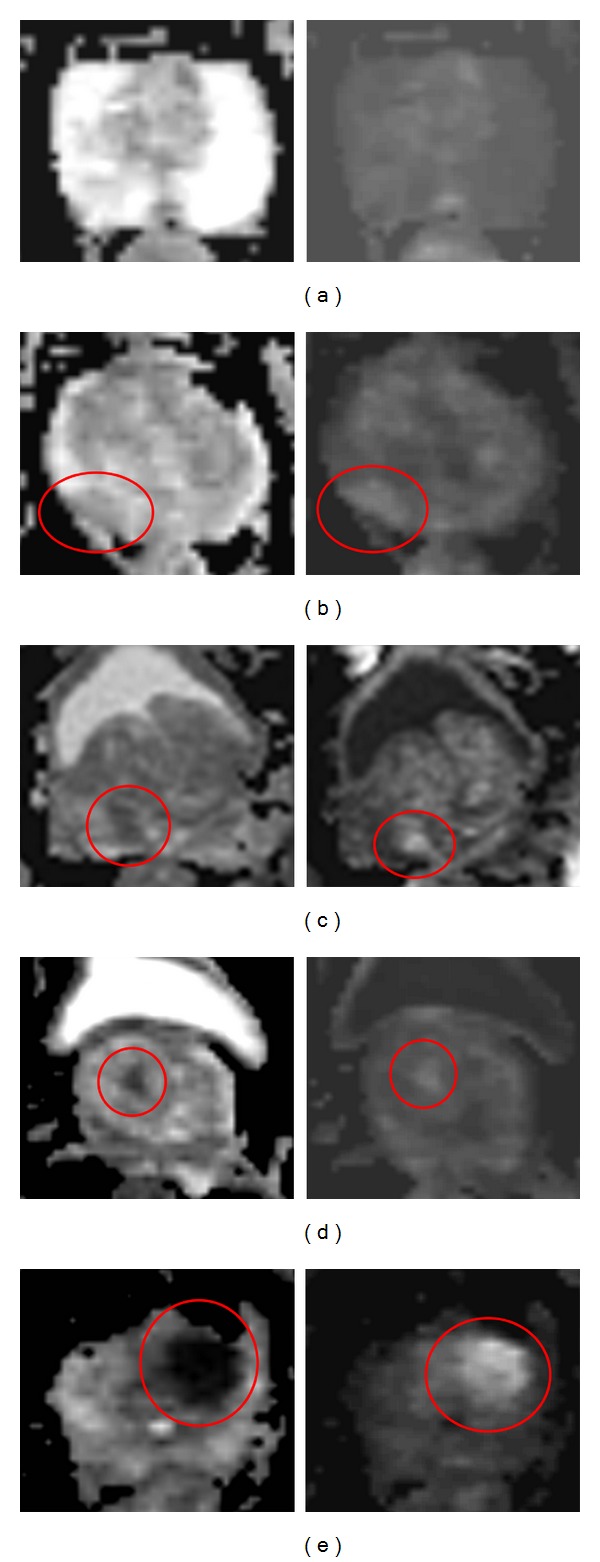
Examples of PI-RADS scoring for DWI. Left: axial ADC map. Right: axial DWI with calculated *b* = 1400. (a) PI-RADS 1. (b) PI-RADS 2. (c) PI-RADS 3. (d) PI-RADS 4. (e) PI-RADS 5.
